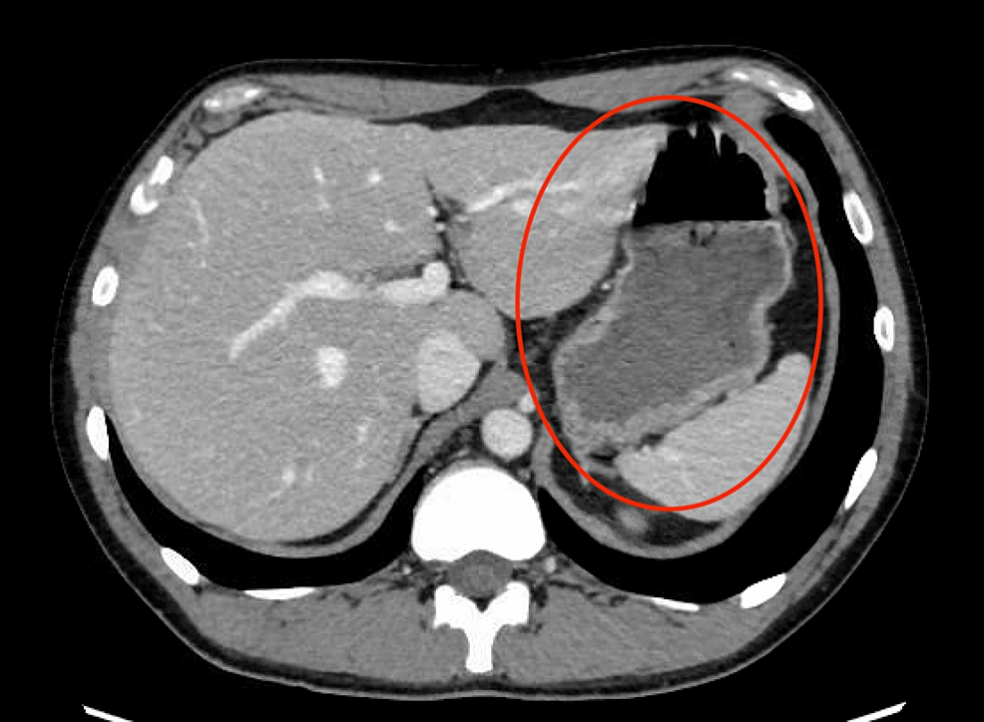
Nonspecific fluid in the left colon An abdominal/pelvis computed tomography (CT) with contrast revealed nonspecific fluid in the left colon.
